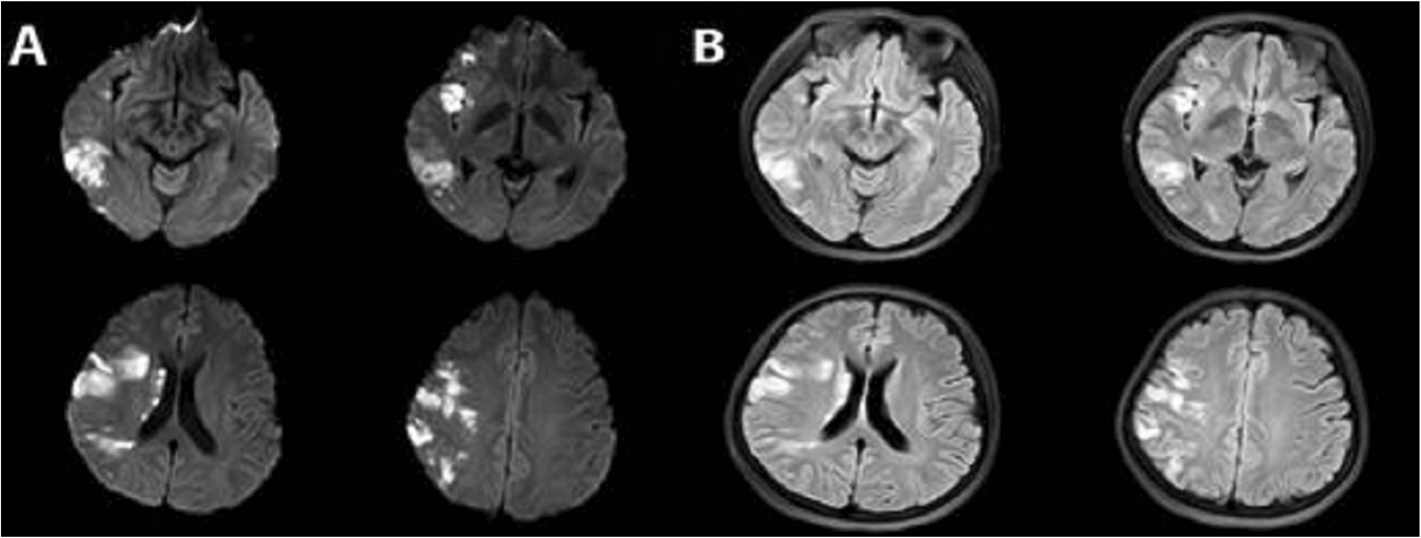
The MRI after two days of onset. Acute cerebral infarction in subcortical of right frontotemporal lobe and basal ganglia. a Diffusion weighted imaging (DWI). b Fluid-attenuated inversion recovery (FLAIR)
